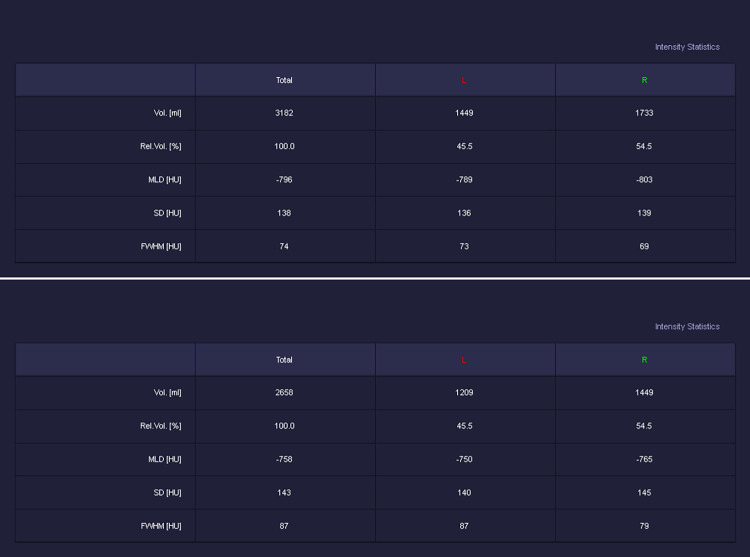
Example of volumetry and densitometry parameter measurements using the 3D chest CT of a representative subject in the A) inspiratory and B) expiratory phases.
